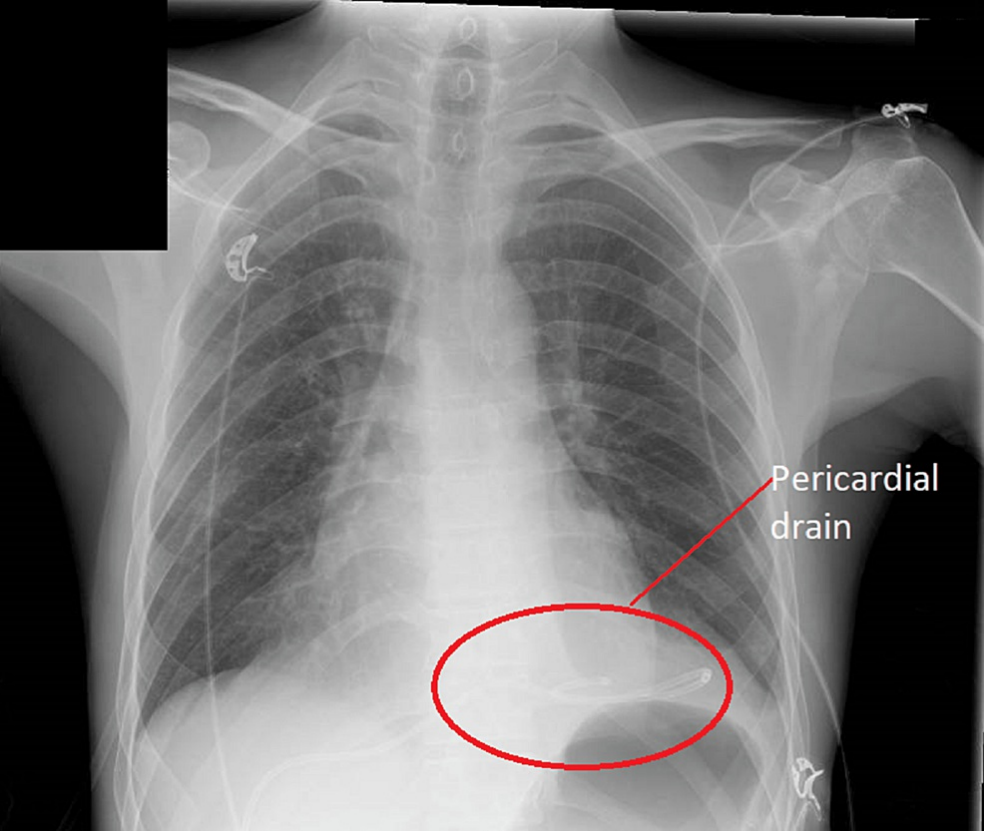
Chest X-ray Significant improvement in cardiac shadow after draining. Pericardial drain in situ.
